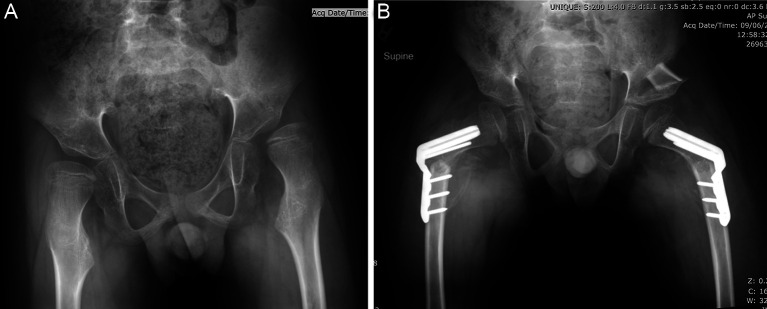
(A) Preoperative radiograph of 9-year-old child with Cerebral Palsy, GMFCS IV with progressive left hip subluxation. (B) Six-week postoperative radiograph demonstrating bilateral femoral osteotomies with cannulated blade plate and a pericapsular osteotomy of Dega on the left side using a wedge of bone from the femur.
